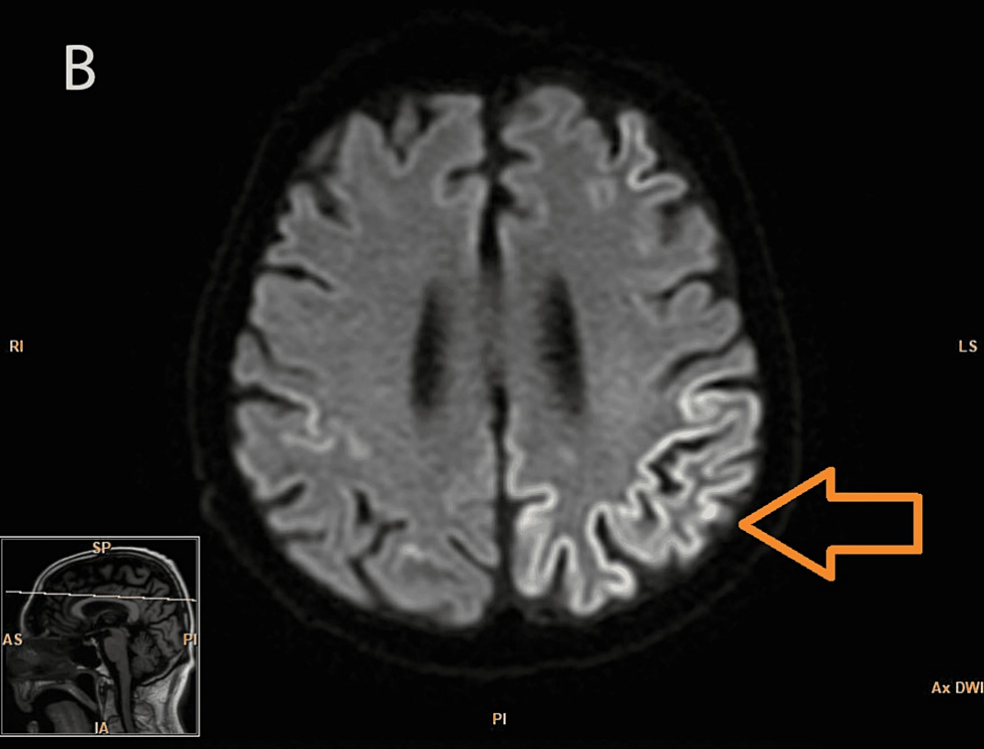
Axial diffusion-weighted MRI showing hyperintensities Abnormal high signal intensities in the occipital and parietal regions of the cortex (arrow)
